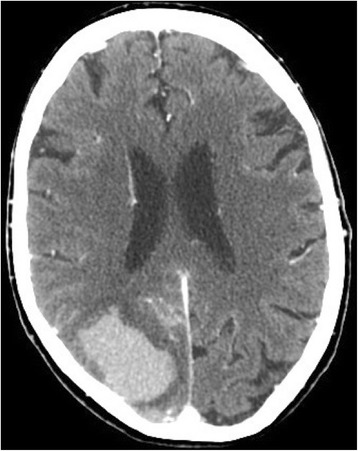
Axial CT angiogram demonstrating acute hemorrhage to the right occipitoparietal region, with surrounding edema
